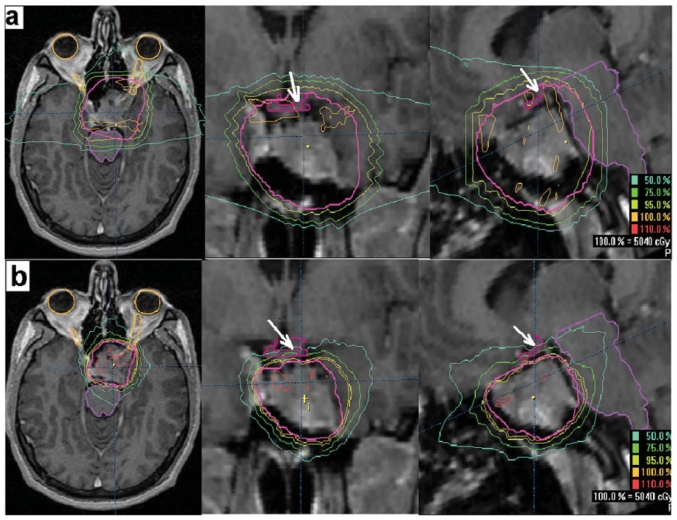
Axial, coronal, and sagittal magnetic resonance imaging slices with isodose distributions from (a) conventional 3-field technique, showing coverage of the planning target volume (ptv) and optic chiasm by the 95% isodose surface; and (b) 5-field fractionated stereotactic radiotherapy technique showing coverage of the ptv by the 100% isodose surface, while keeping the optic chiasm (arrow) outside the 75% isodose surface.
